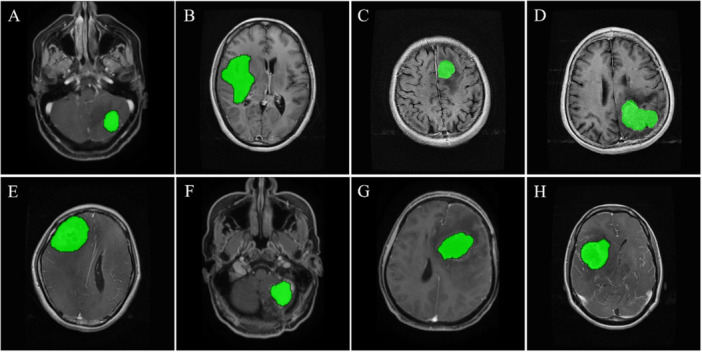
Representative contrast‐enhanced T1‐weighted (T1Gd) MRI images from randomly selected patients with high‐grade gliomas (HGGs). The manually tumor regions of interest (ROIs), used to generate volumes of interest (VOIs) for radiomics feature extraction, are overlaid in green.
